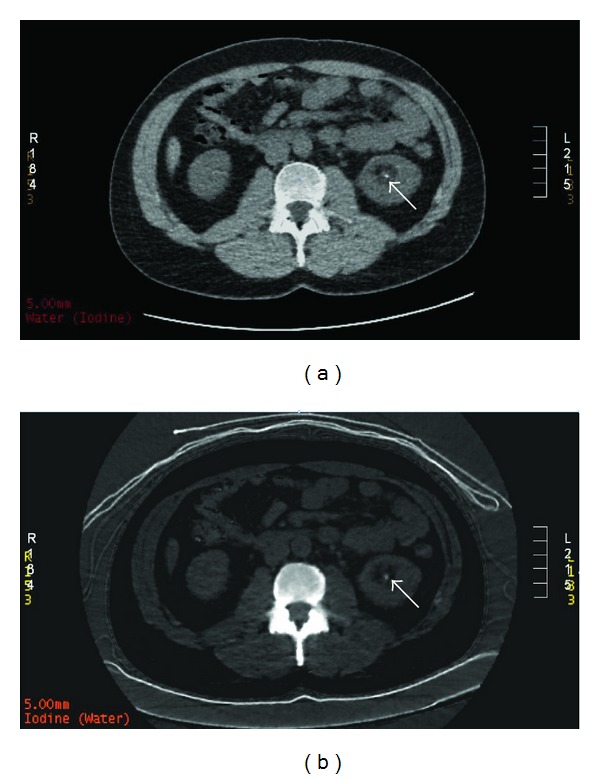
A 34-year-old male presenting with left flank pain. Axial dual energy noncontrast CT. (a) shows a 3 mm calculus in the left renal pelvis on iodine and (b) water-based attenuation. As the calculus is visible on both imaging techniques, this indicates high-molecular-weight elements. This calculus proved to be predominantly calcium based.
